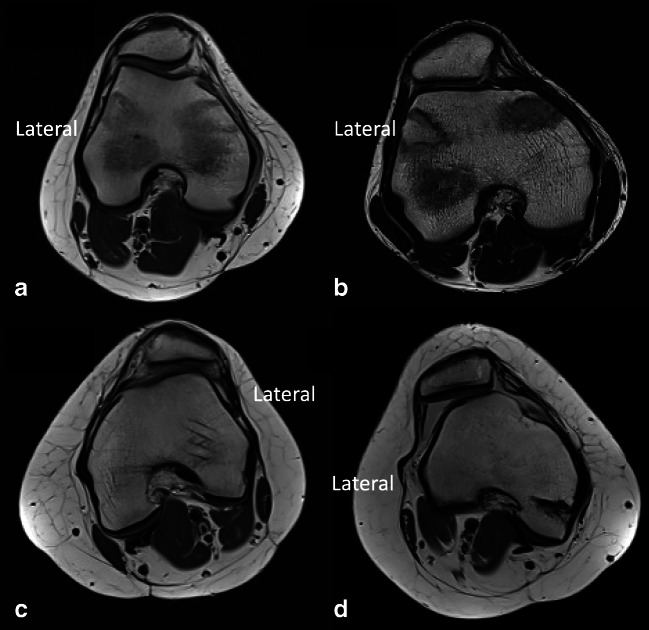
Examples of trochlea dysplasia evaluated on axial MRI. Evaluation is performed on the most proximal axial image with the complete cartilaginous trochlea. a Type A in a right knee with a shallow trochlea. b Type B in a right knee with a flat to convex trochlea. c Type C in a left knee with asymmetry of the trochlear facets and hypoplastic medial condyle. d Type D in a right knee with asymmetry of the trochlear facets and the medial facet appears elevated. Note that the patella is laterally dislocated.
